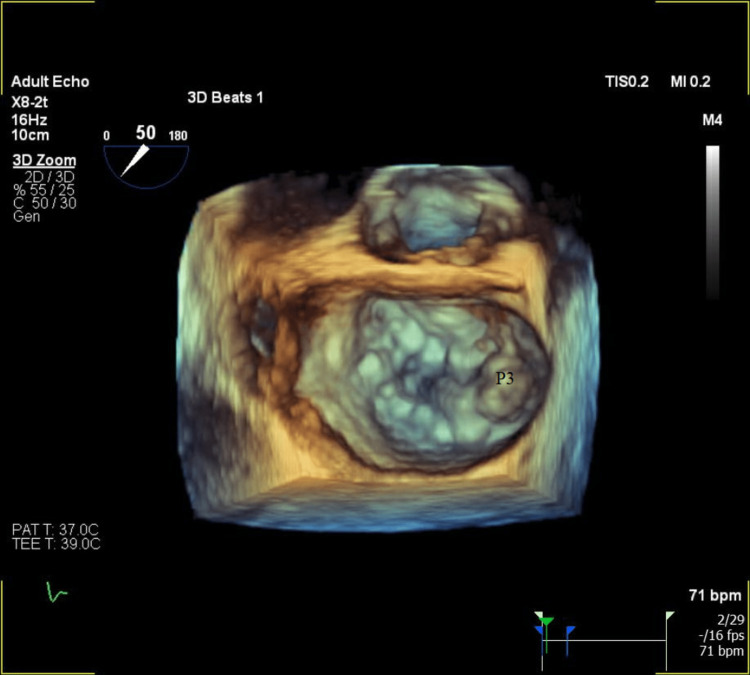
Preoperative transesophageal echocardiogram multiplanar 3D reconstruction of the mitral valve, en face view, showing the flail P3 segment during systole P3: flail P3 segment
